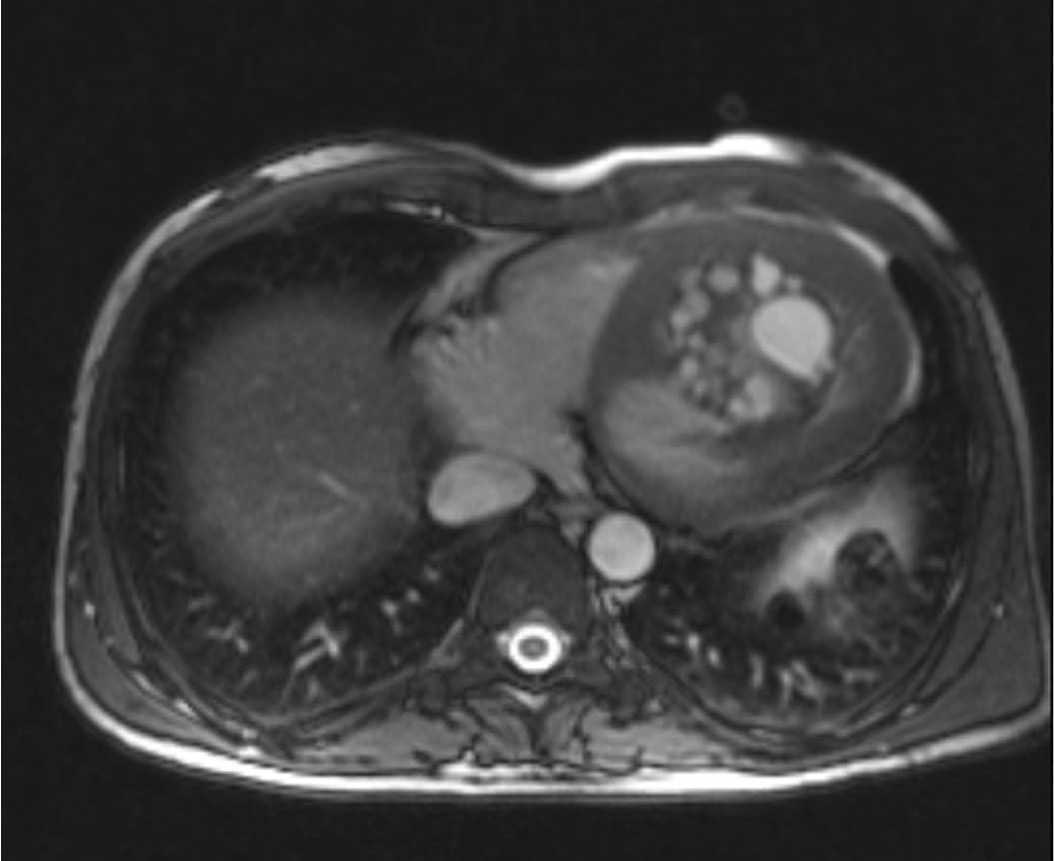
Axial T2‐weighted CMR of a 35‐year‐old male with multi‐organ hydatid cyst. There is an intracavitary multiloculated cystic lesion with a high signal content of cysts and a thin hypo‐signal wall occupying a large part of the lumen of the LV. CMR, cardiac magnetic resonance imaging; LV, left ventricle.
